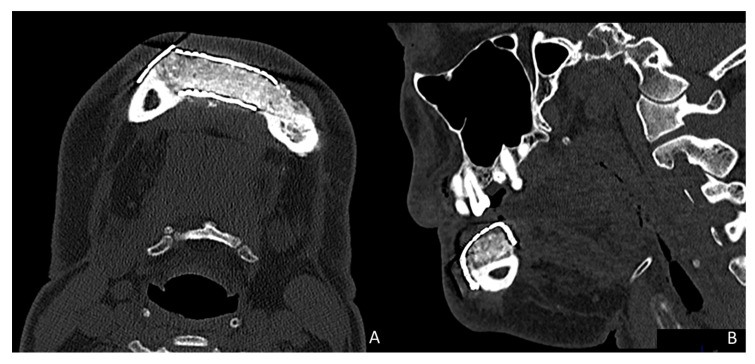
Postoperative CT scan. (A) Axial CT Scan demonstrating the stability of the transverse dimension of the fibula with respect to the remnant mandible. (B) The three-dimensional preservation of the iliac crest graft with CAD/CAM mesh makes it possible to double the height of the fibula.
